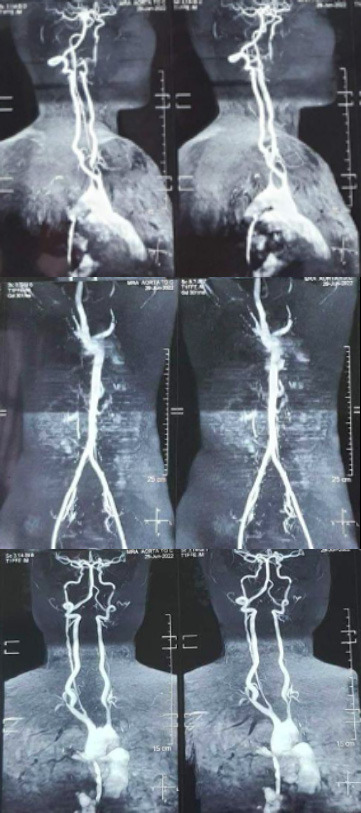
MRI scan showing thickening of the arch of the aorta and narrowing of the lumen of bilateral subclavian arteries.
